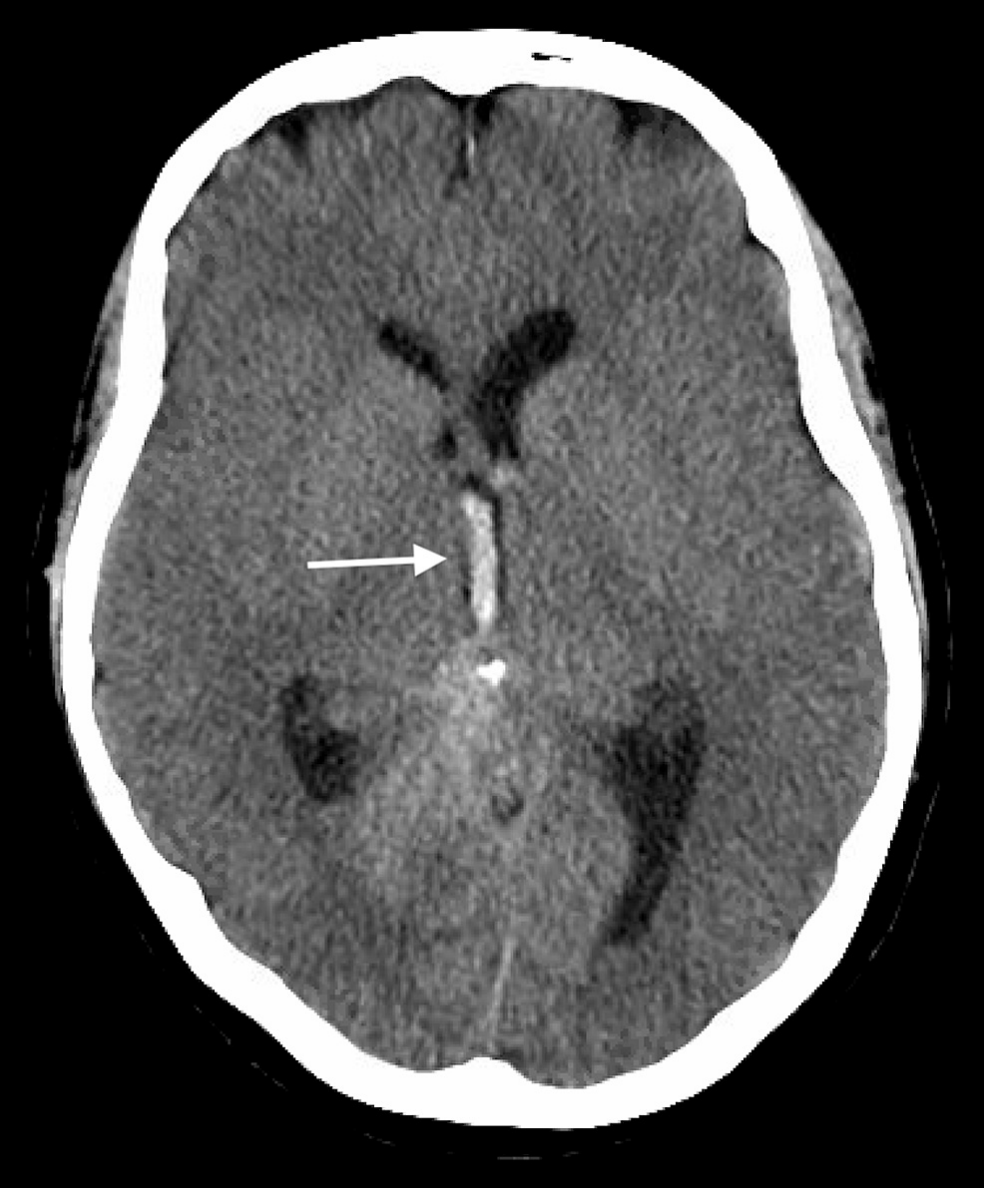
Axial non-contrasted CT head with evidence of intra-ventricular hemorrhage within the third ventricle and early ventriculomegaly (arrow). CT, computed tomography.
